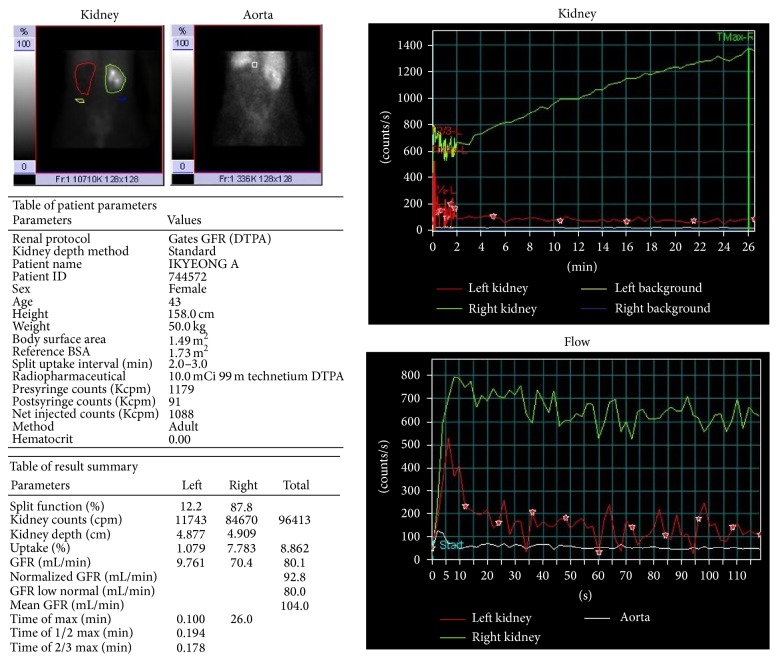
Renal scintigraphy. Complete loss of function of the left kidney.
